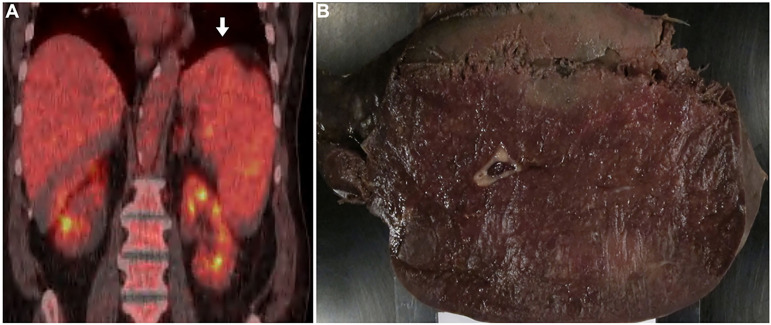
Imaging and gross findings: (A) PET/CT scan showing splenomegaly with diffuse mild homogenous uptake (white arrow). (B) Gross image of the spleen showing splenic congestion with no obvious lesions or masses.
